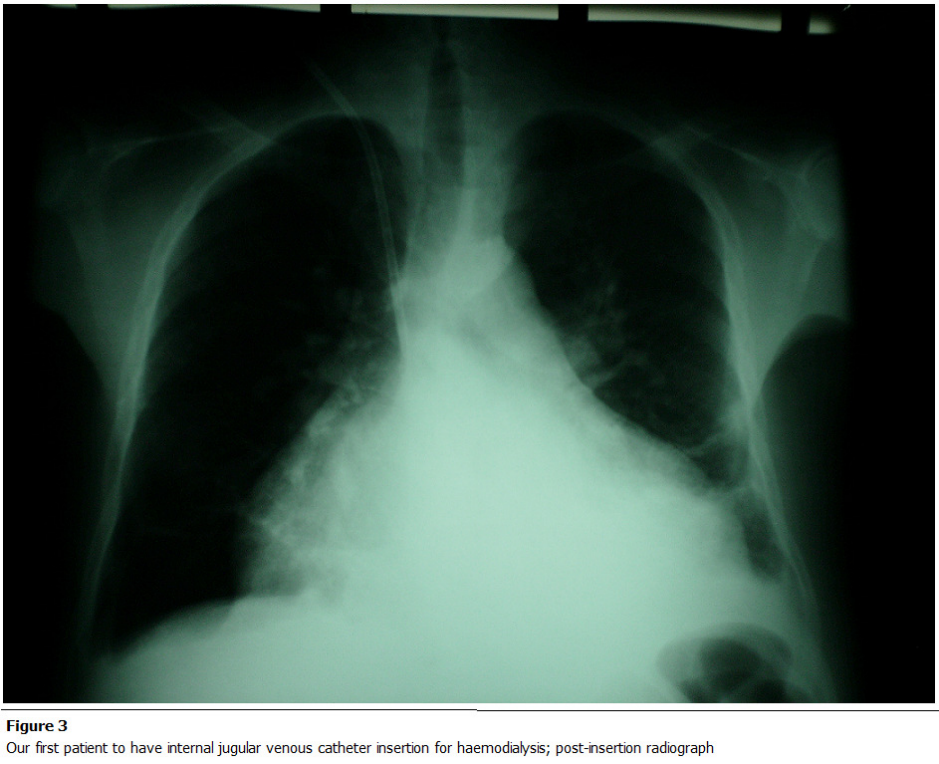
Our first patient to have internal jugular venous catheter insertion for haemodialysis; post- insertion radiograph
